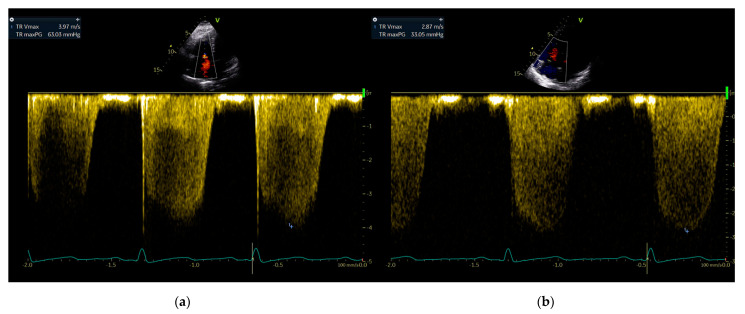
Echocardiogram in a patient with a high-altitude dweller, showing an exaggerated rise in pulmonary artery pressure during the cold season. Peak tricuspid regurgitation jet velocity estimating right ventricular systolic pressure measured in (a) the first examination in late winter, and (b) a repeat examination in late summer.
